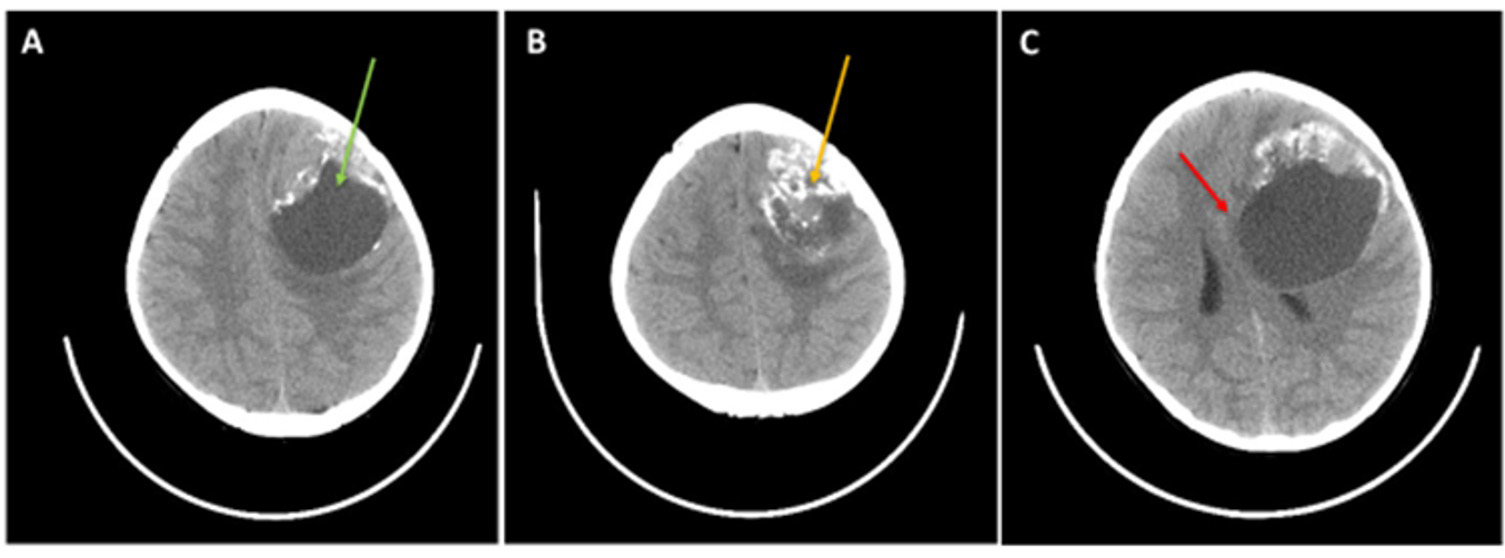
Grade III ependymoma unenhanced computed tomography (CT) of the brain. A large lesion (5.4 × 7.5cm) in the left cerebral frontoparietal location with predominantly cystic components (panel
